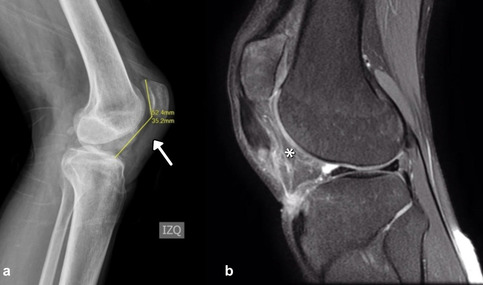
(a) Lateral radiograph at 30° of the left knee showing patella alta (Caton‐Deschamps Index 1.48). (b) MRI (sagittal view) of the left knee confirming patellar tendon rupture (*) without avulsion fractures. (MRI, magnetic resonance imaging.) Position: supine. Operated side: left.
